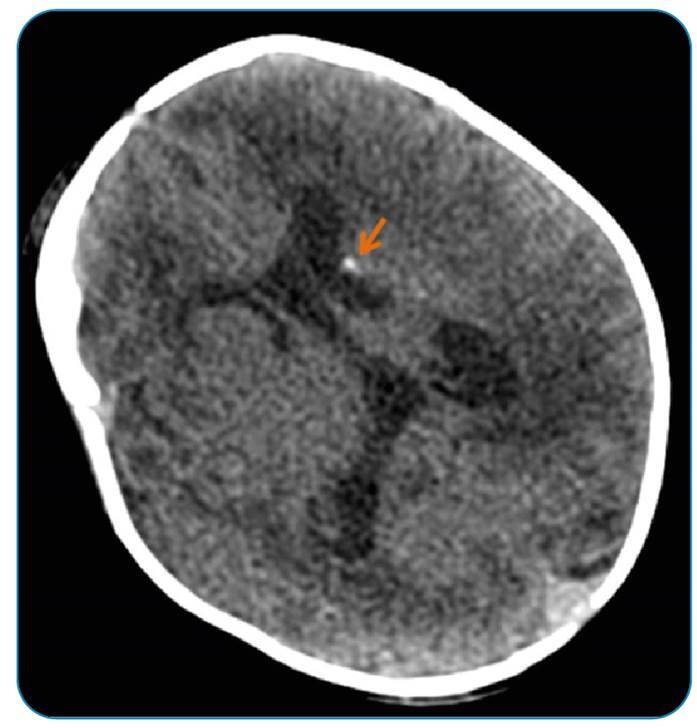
Unenhanced axial computed tomography image shows periventricular calcification (arrow) and hypodense white matter involvement.
